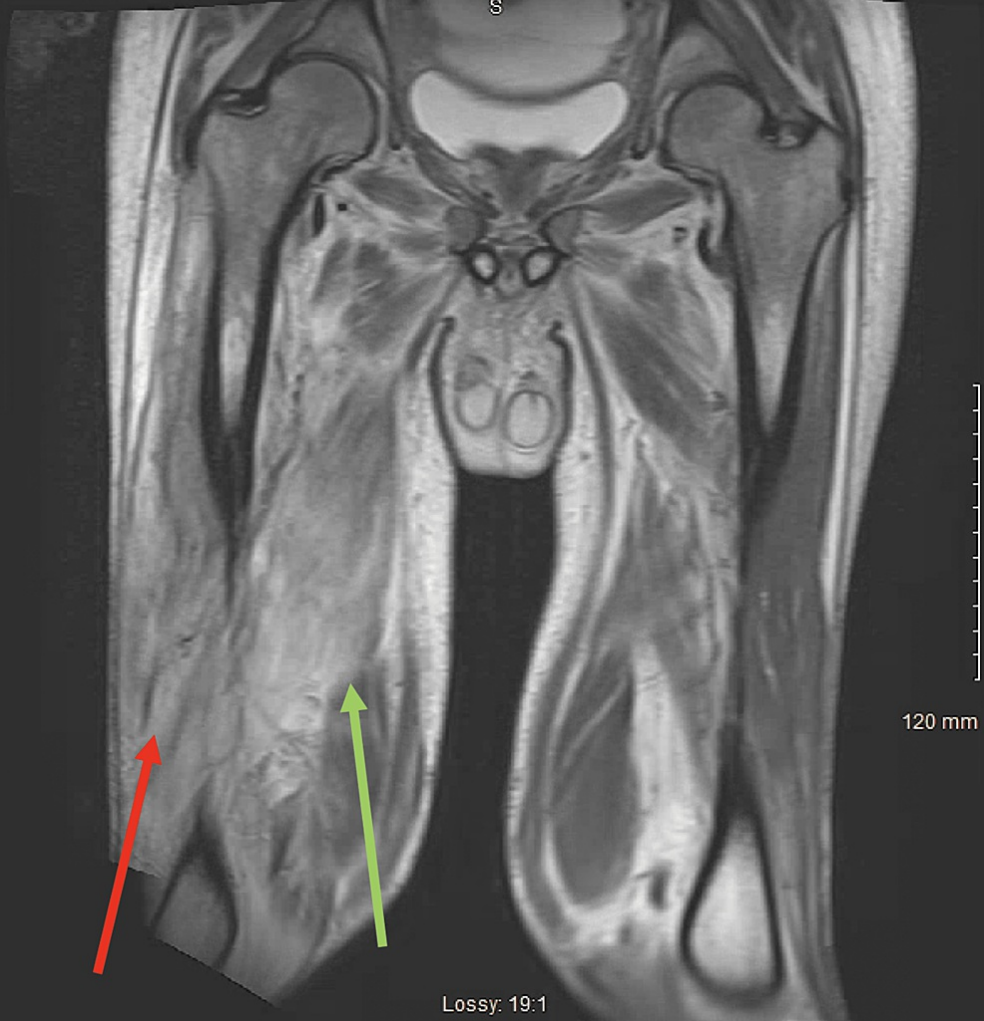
MRI of the right hip with and without contrast. T-2 weighted coronal MRI of the femur showing evidence of muscle edema and muscle enhancement involving the anterior compartment and the abductor muscles, suspicious for myositis. There are irregular areas of non-enhancement within the vastus lateralis (red arrow) and vastus medialis (green arrow) muscles which may represent myonecrosis or developing abscess.
